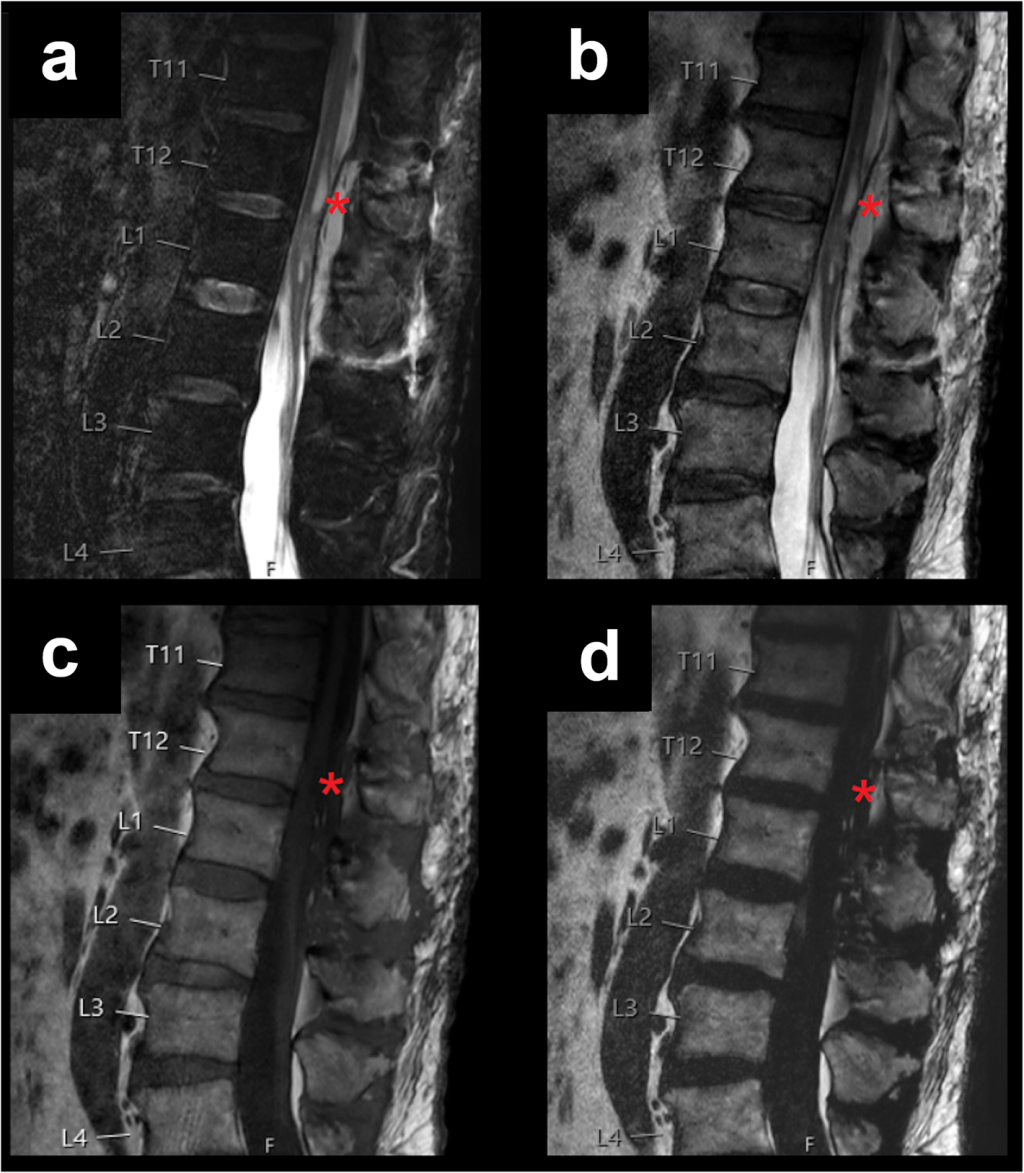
Postoperative thoracolumbar haematoma. This figure shows the lower thoracic and lumbar spine of a 69-year-old male patient using water-only images (a), in-phase images (b), and fat-only images (d) of the sagittal T2-weighted DIXON sequence, together with a sagittal T1-weighted sequence (c). The patient had undergone decompressive lumbar surgery and presented with a postoperative epidural haematoma between T12 and L2 (red asterisks)
